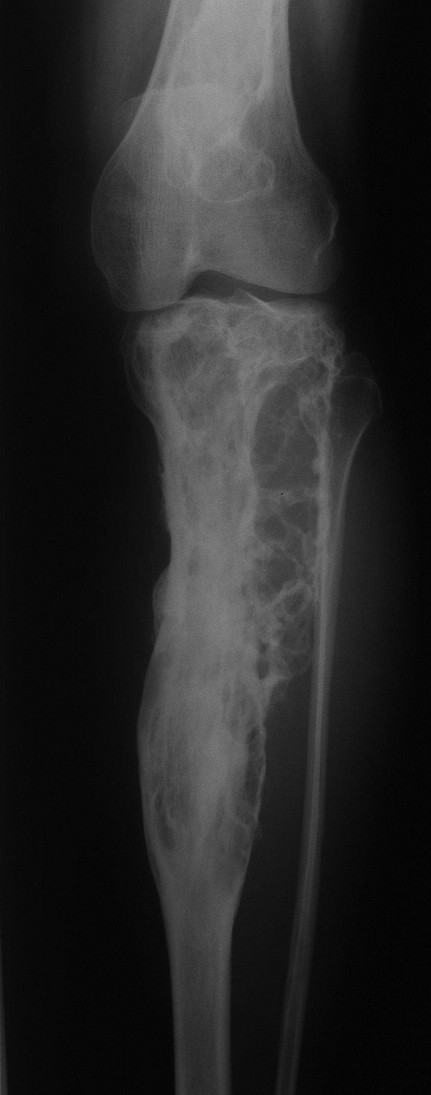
X-ray of the left tibia. Anteroposterior plain film of the left tibia showing fibrous dysplasia.
